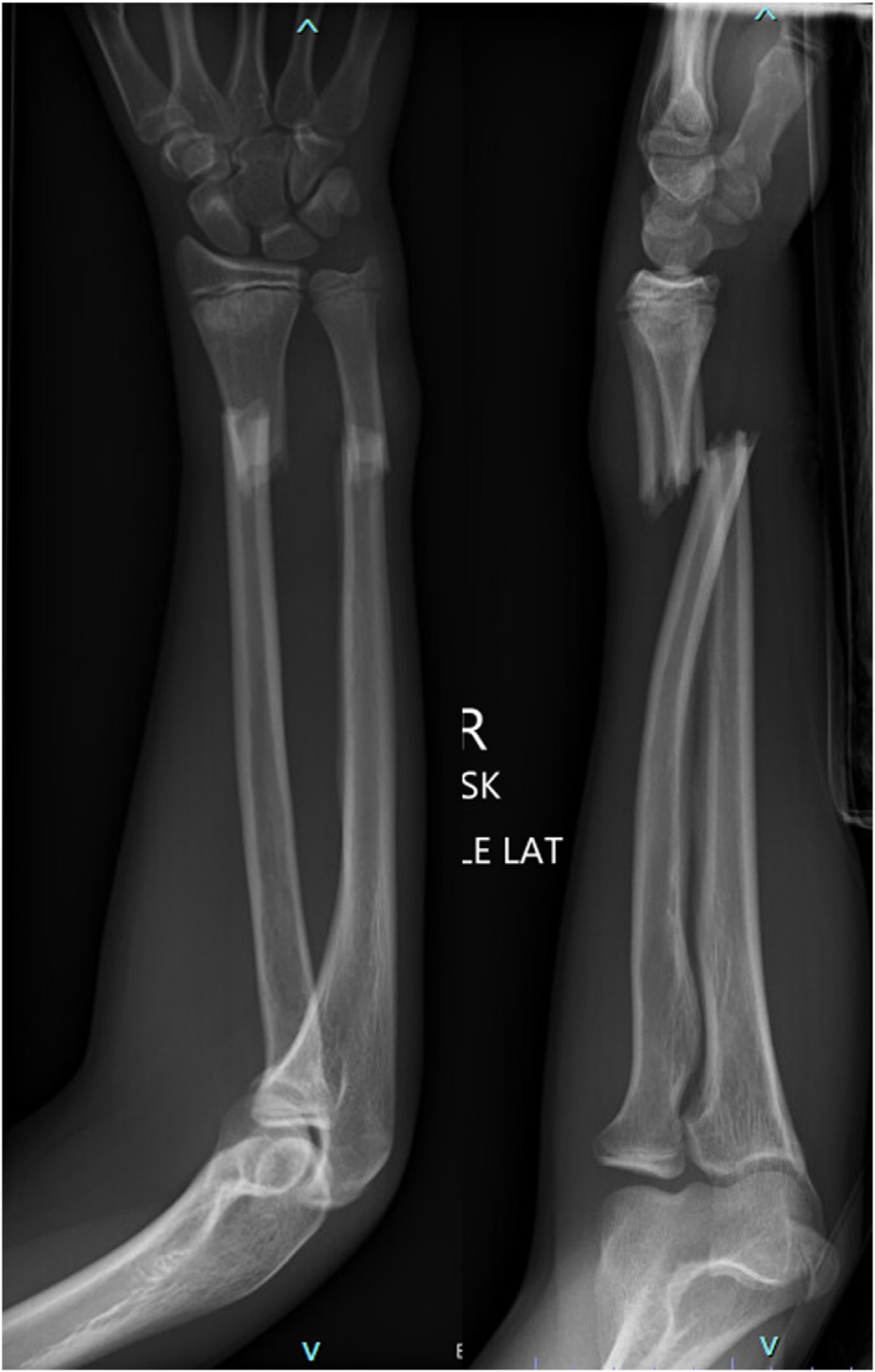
Lateral and poster–anterior radiographs at the time of injury demonstrating a distal one-third radial and ulnar shaft fracture with significant dorsal displacement and overlap of the fragments.
